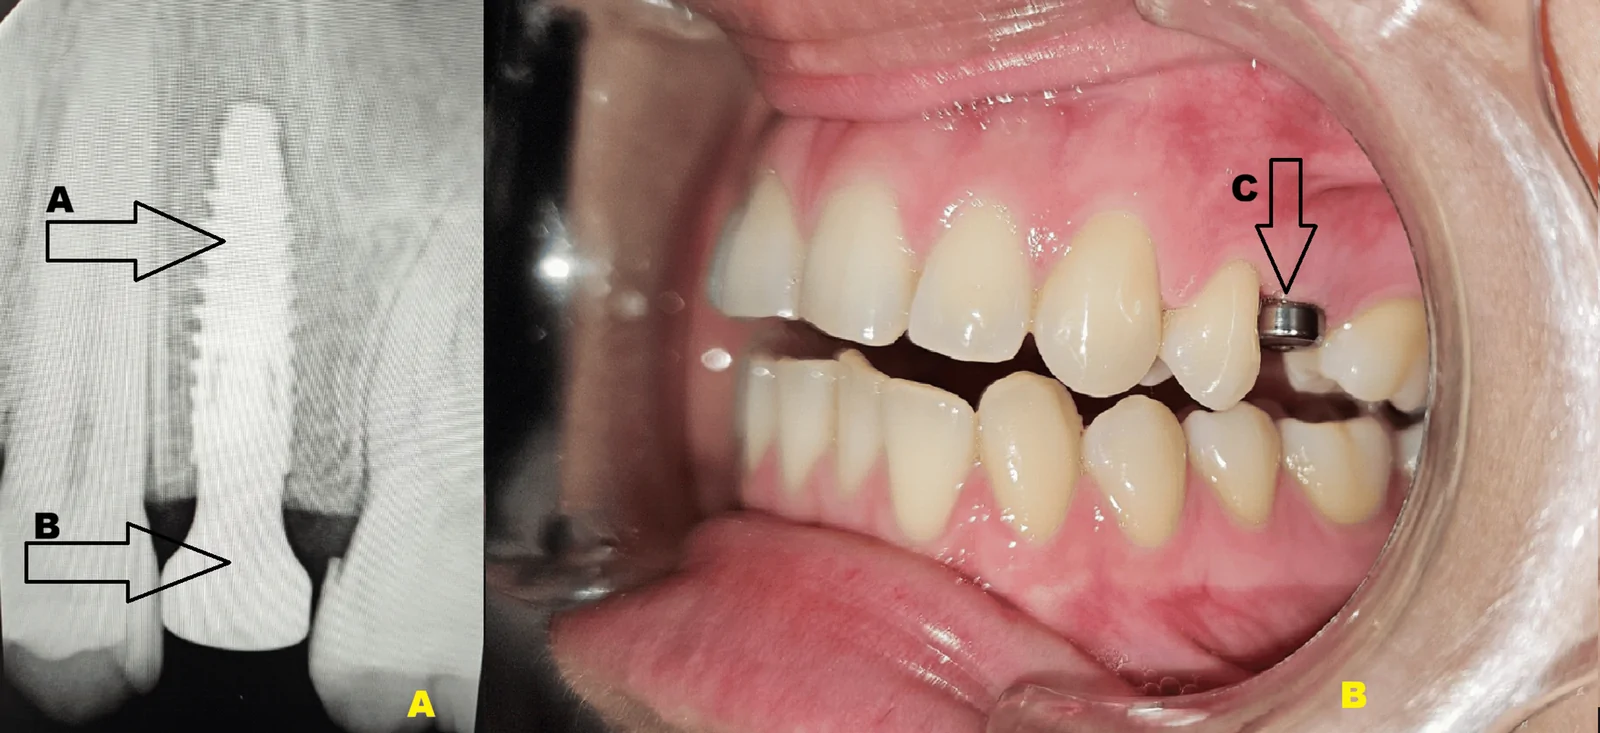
Initial radiographic and clinical presentation of the symptomatic maxillary implant (A) Periapical radiograph showing the implant fixture in the left posterior maxilla. (B) Implant abutment connected to the implant after loss of the implant-supported crown. (C) Clinical view of the implant site demonstrating the exposed abutment following crown loss.
